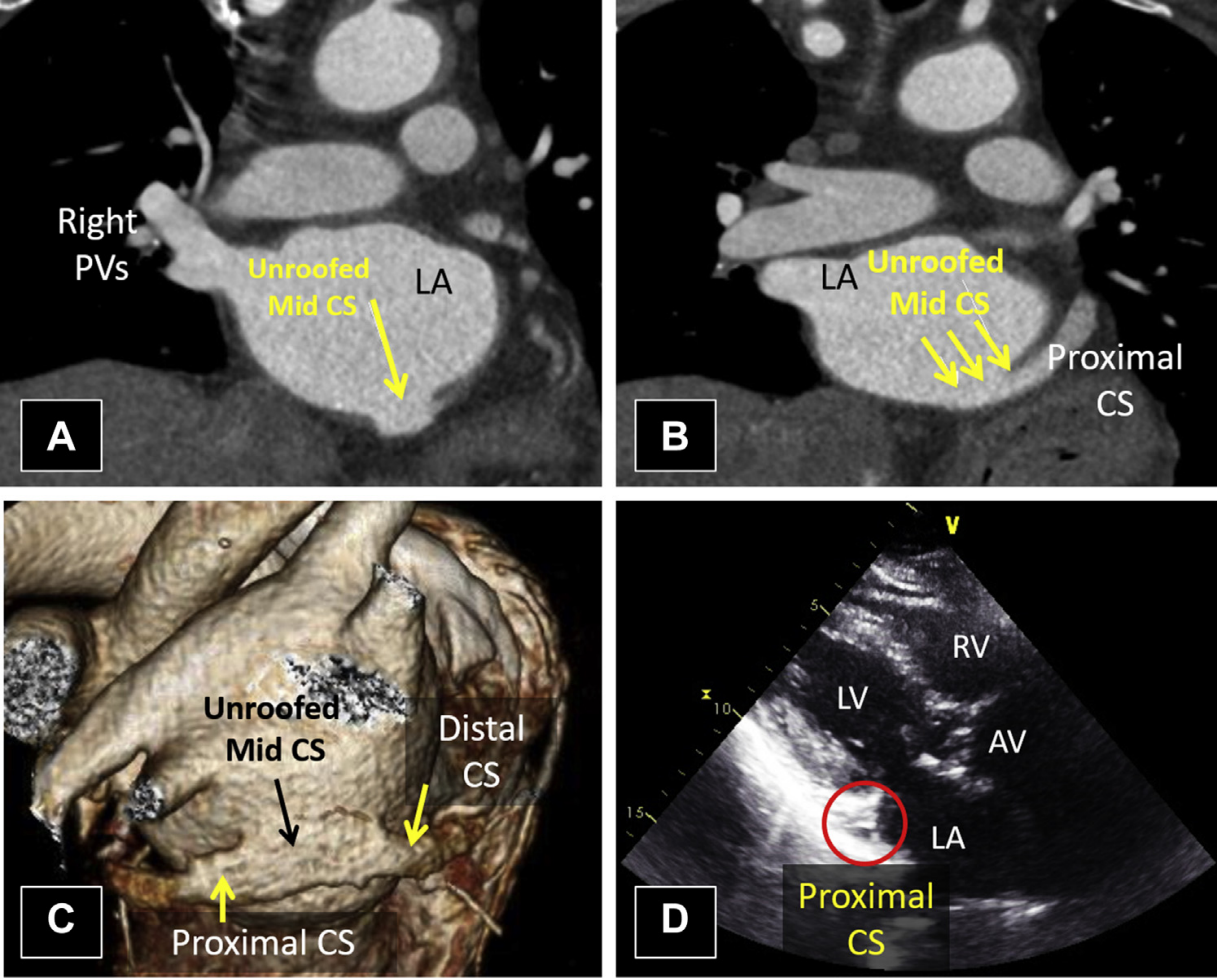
Case 4: unroofed CS without persistent L-SVC. Cardiac CT imaging with coronal sections (A, B) and three-dimensional rendering (C) and TTE in the parasternal long-axis view. The lack of a discrete division between the LA and the middle segment of the CS indicates an unroofed mid CS (A-C). While the connection between the CS and LA is seen on CCT imaging, the CS appears normal and nondilated on TTE (D). This is because TTE visualized the proximal segment of the CS. Video 5 corresponds to this figure. *AV*, Aortic valve; *CS*, coronary sinus; *PV*, pulmonary vein; *RV*, right ventricle.
